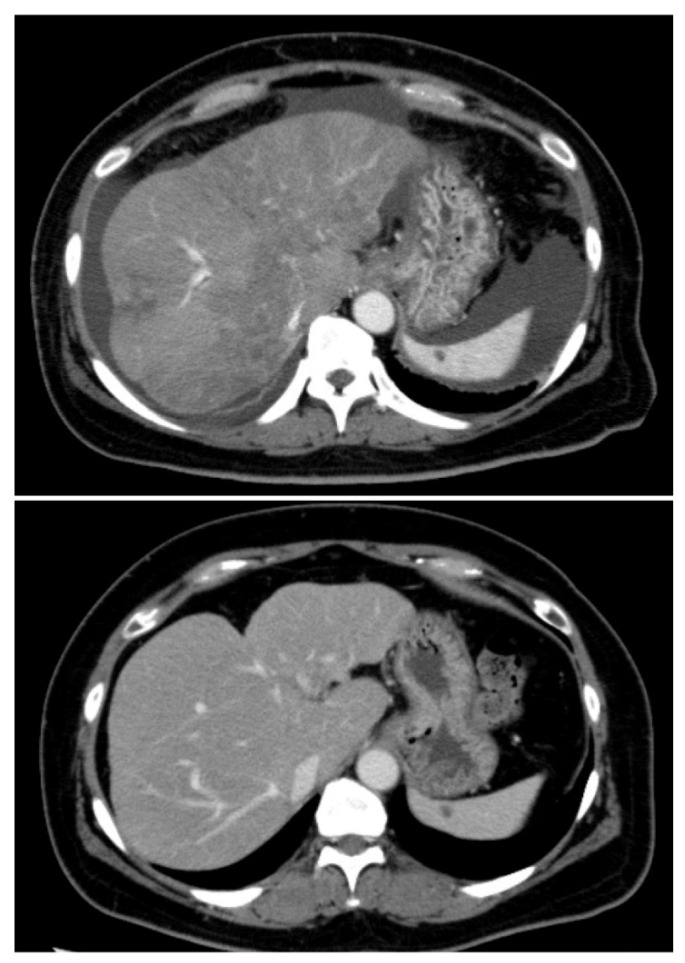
Computed tomography performed before (above) and after (below) alectinib therapy. Liver lesion and ascites disappeared after alectinib therapy.
